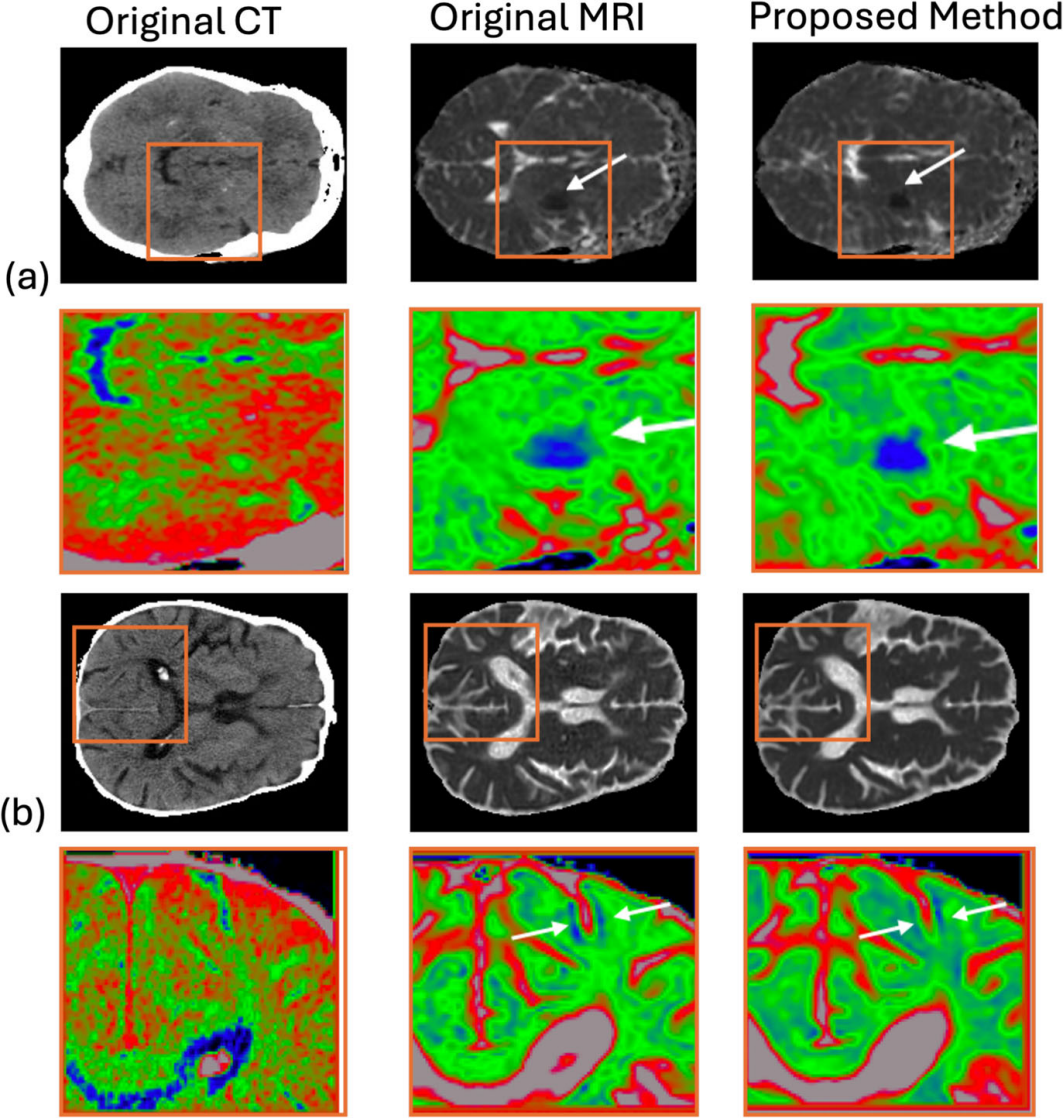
Example of low ADC signal lesions on MRI in a left basal ganglia and b cortex of two test cases with acute infarct lesions on original MRI and the proposed synthetic images derived from essentially normal CT. To improve contrast for visual examination, Rainbow colour maps (bottom row for each case) were generated using ImageJ, where blue represents low signal strength and red indicates high signal strength
